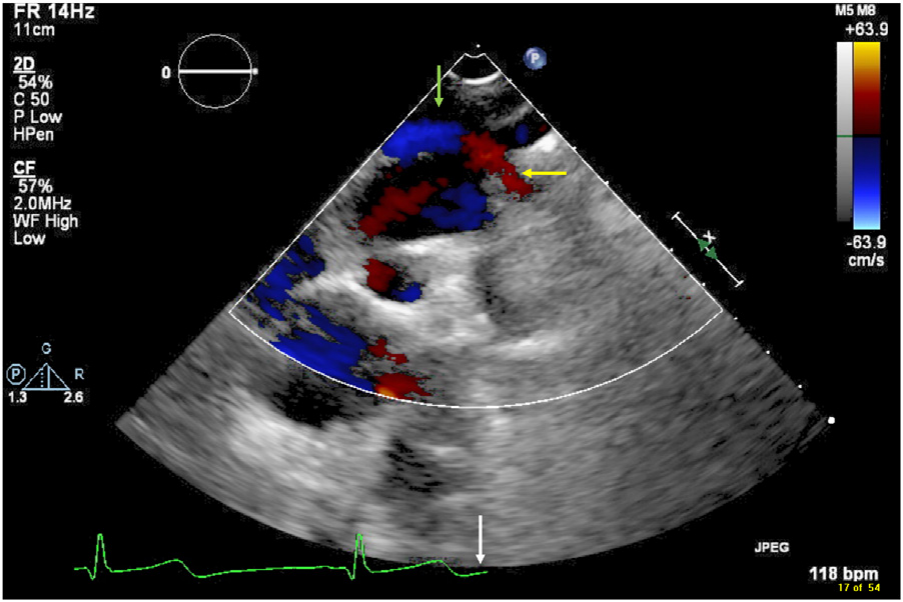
Two-dimensional TTE with color flow Doppler, suprasternal sagittal view, diastolic phase *(white arrow)*, demonstrates mild dilation of the innominate vein *(green arrow)* with red color flow suggesting an anomalous vessel *(yellow arrow)* coursing cephalad to enter the dilated innominate vein.
